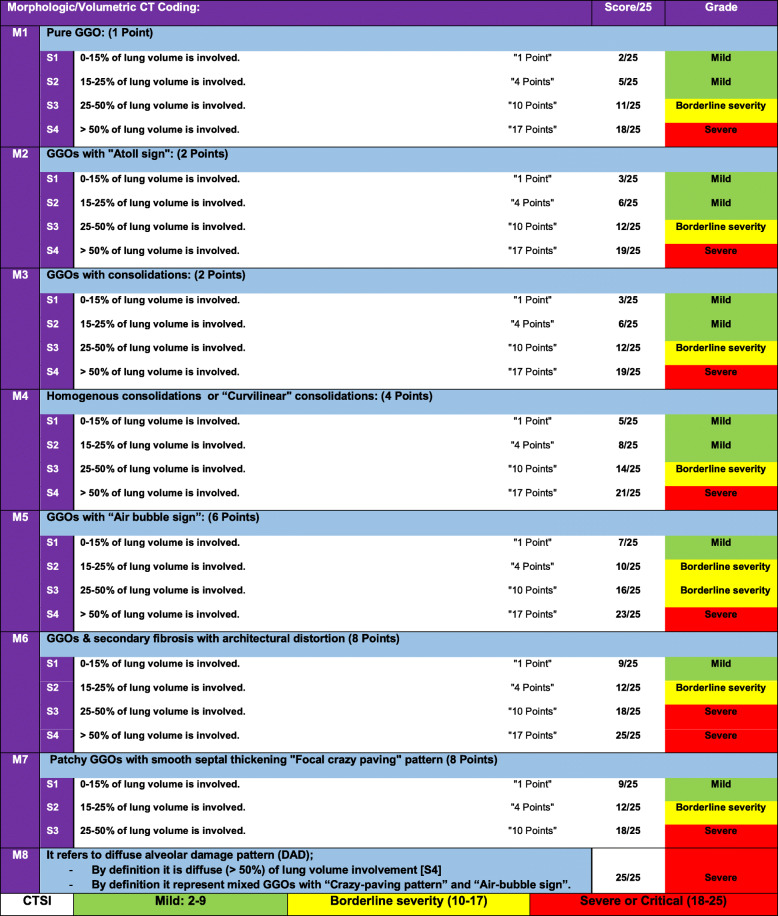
Combined morphologic/volumetric CT coding—new proposed CTSI for COVID-19
